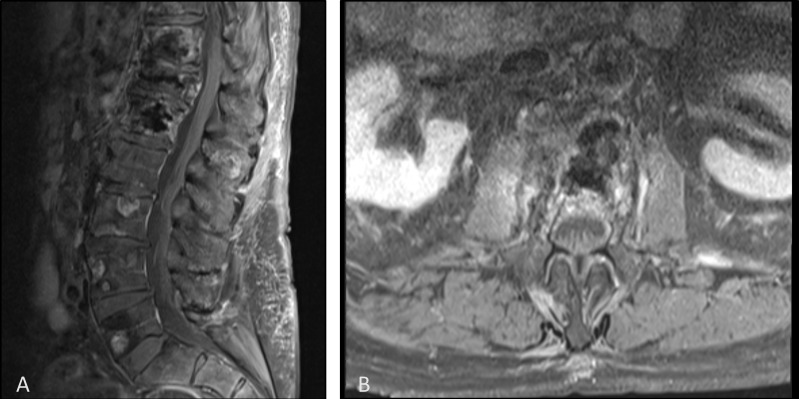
Post-treatment contrast enhanced T1 weighted magnetic resonance imaging of the sagittal spine (A) and axial spine at L1 (B) showing vertebral augmentation changes and resolution of epidural disease and spinal compression
